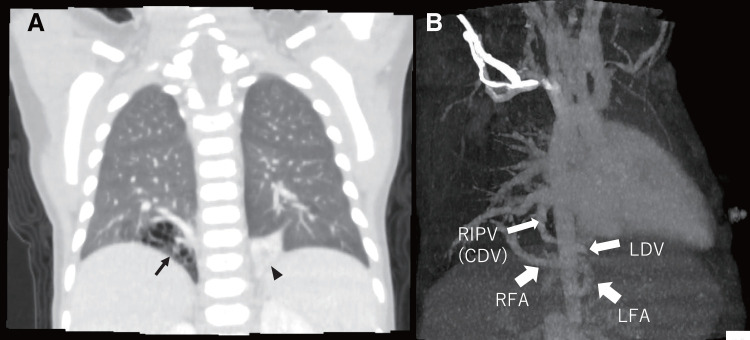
(A) Chest CT scan: Coronal section. The arrow indicates the right IPS, and the arrowhead indicates the left EPS. (B) 3D reconstruction image of CT scan: Feeding arteries and drainage veins in this patient. CDV, common drainage vein; EPS, extralobar pulmonary sequestration; IPS, intralobar pulmonary sequestration; LDV, left drainage vein; LFA, left feeding artery; RFA, right feeding artery; RIPV, right inferior pulmonary vein
